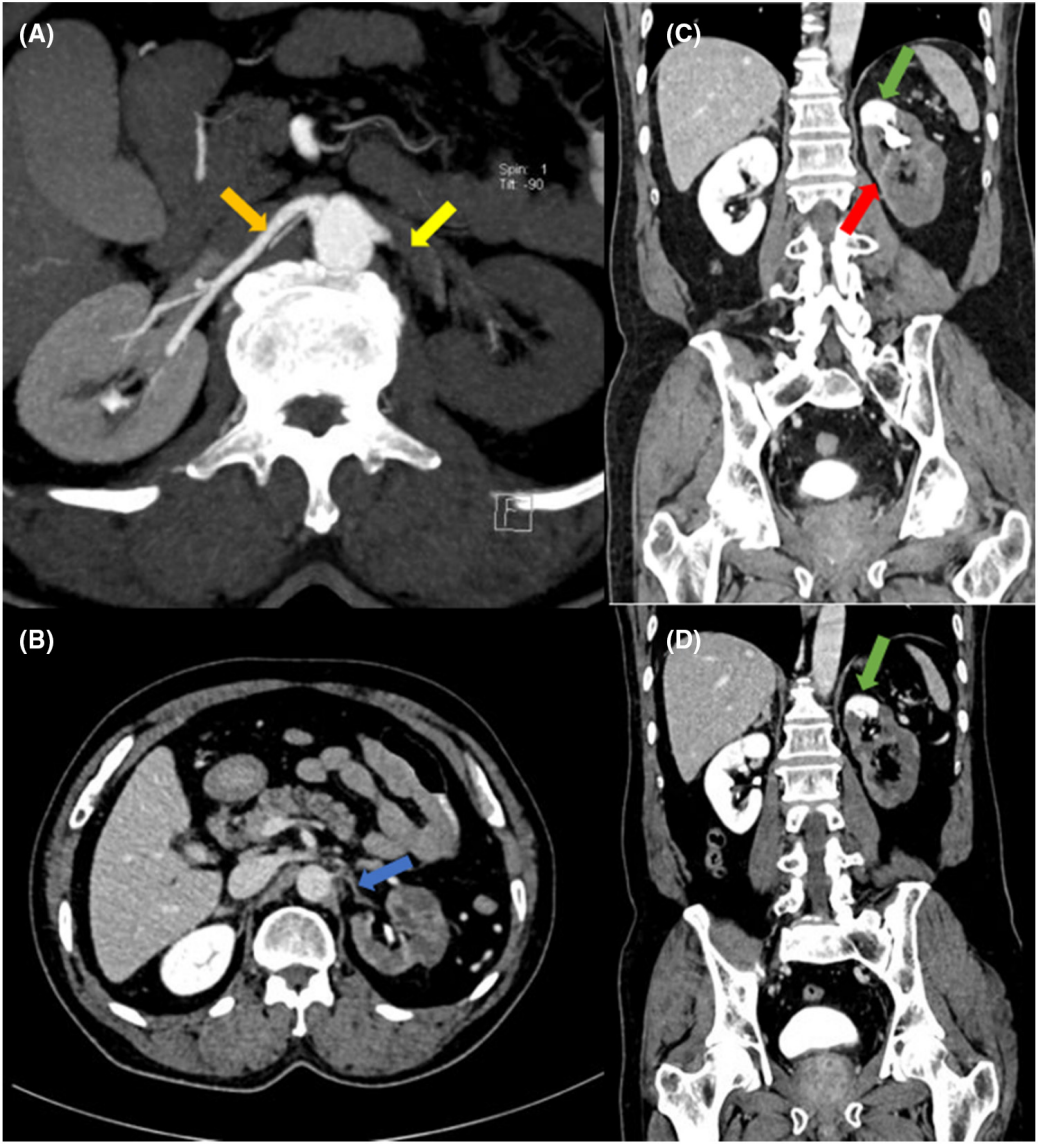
CT angiogram of renal arteries (A) Axial maximum intensity projection, (B) Axial and (C, D) Coronal cuts showing non‐opacification of left main renal artery just beyond its origin (Yellow arrow) with hypoperfusion of left kidney, and faint peripheral enhancement of left kidney (Red arrow). An accessory left renal artery was seen arising from the aorta (Blue arrow) supplying the upper pole of left kidney (Green arrows). The right renal artery is patent (Orange arrow).
